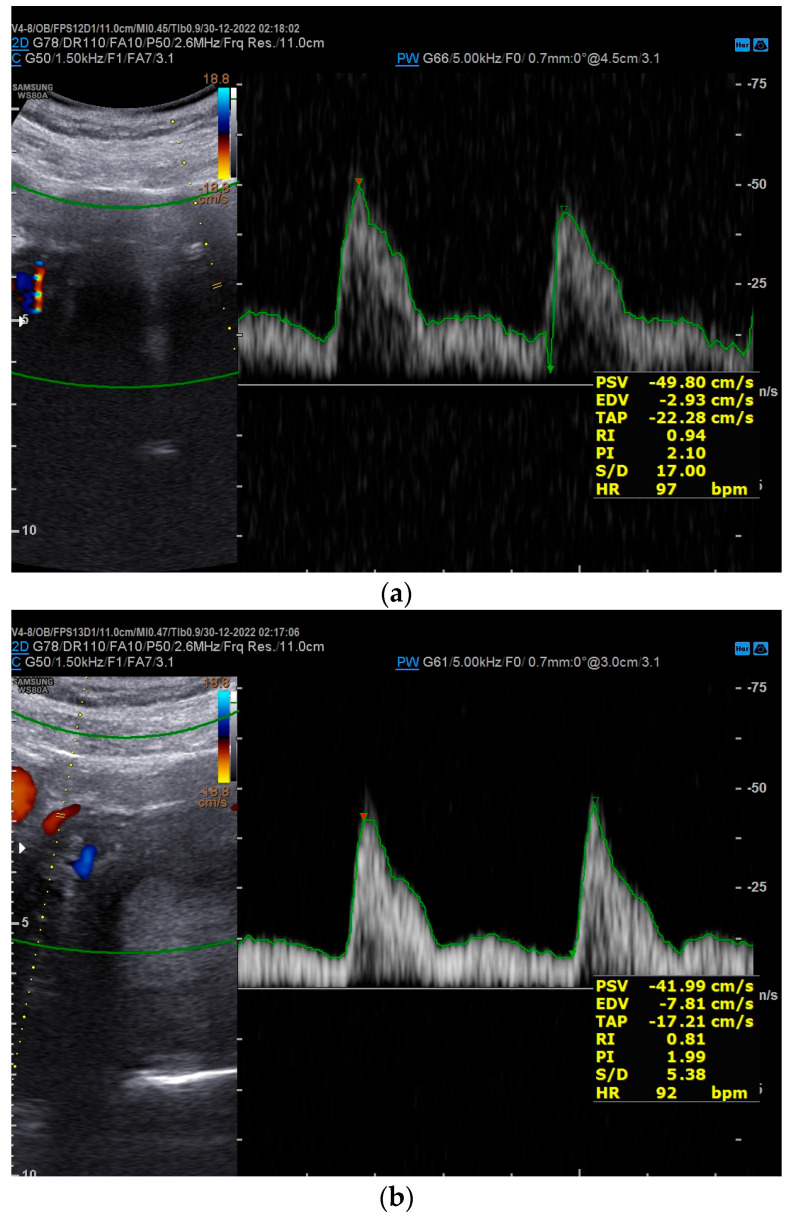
(a,b) Increased resistance in the maternal vessels, both with nodules, in second-trimester ultrasound.
